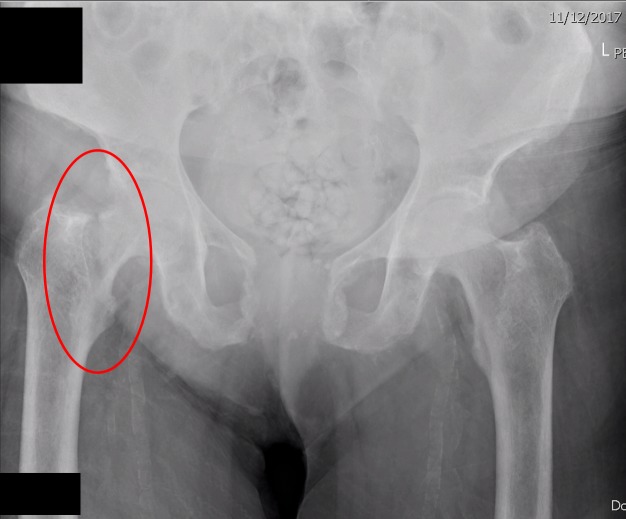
X-ray of the pelvis - basicervical fracture X-ray of the antero-posterior pelvis revealing abnormal bone texture in the right proximal femur with a cortical breach superiorly in the femoral neck.
